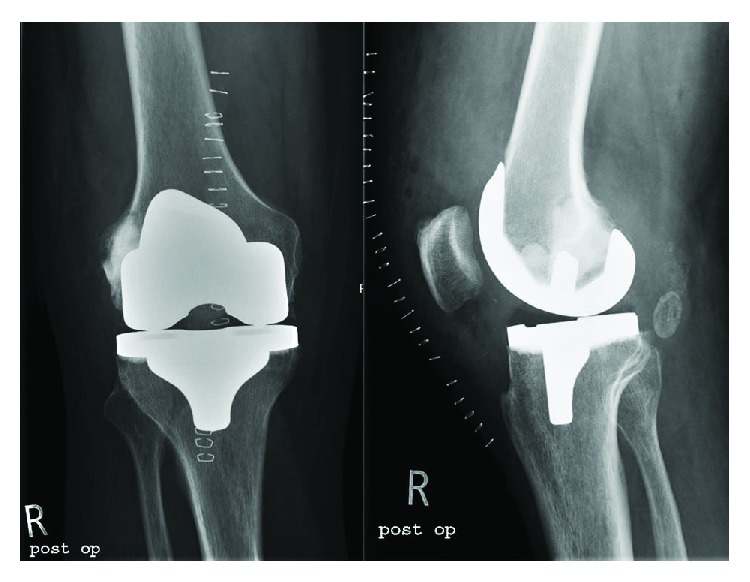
Postoperative radiographs of the right knee confirmed the correct position of the prosthesis and a proper filling of the large bone cyst with Cerament.
